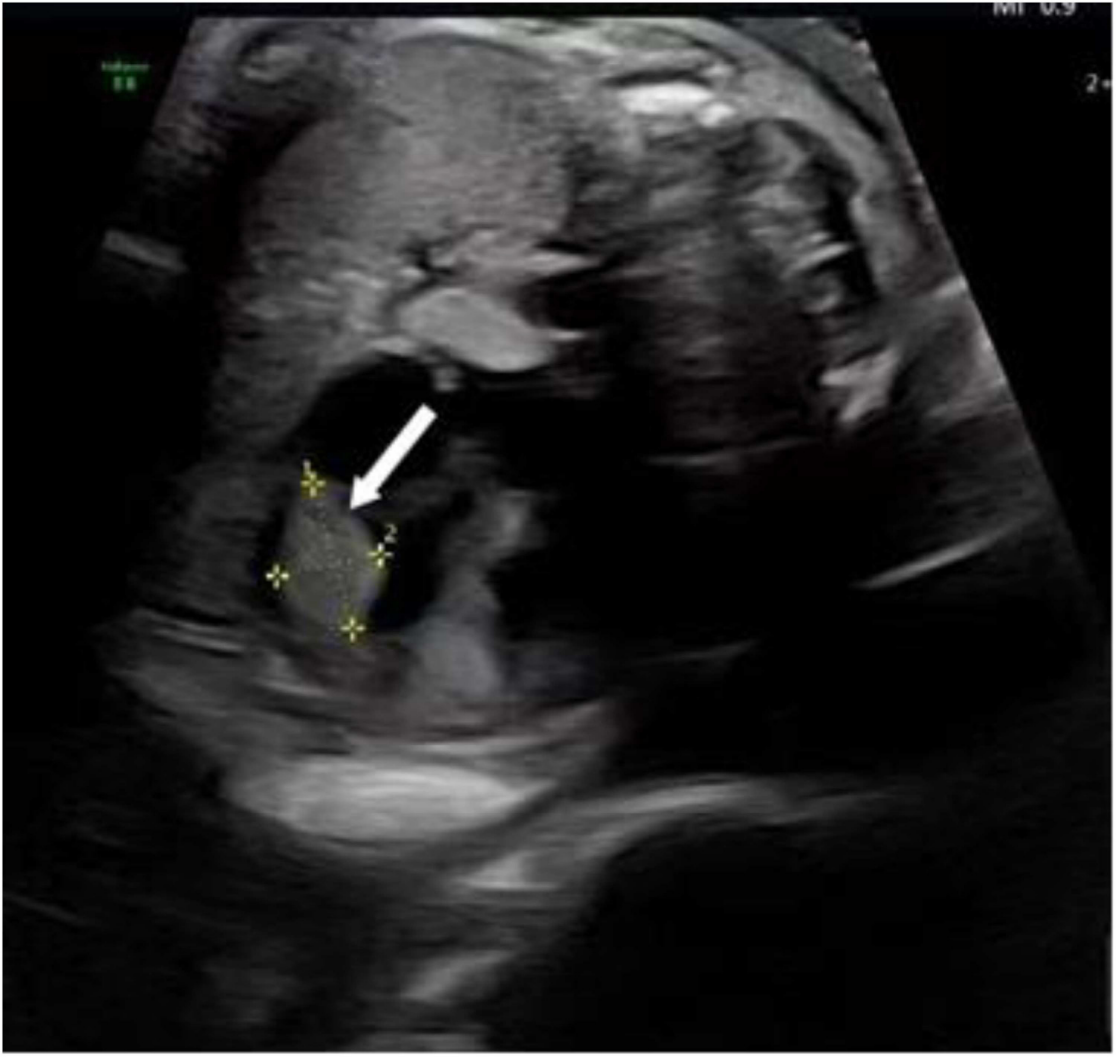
Ultrasonography reconfirmed rhabdomyoma near the apex of the fetal left ventricle, as as indicated by the arrow.
